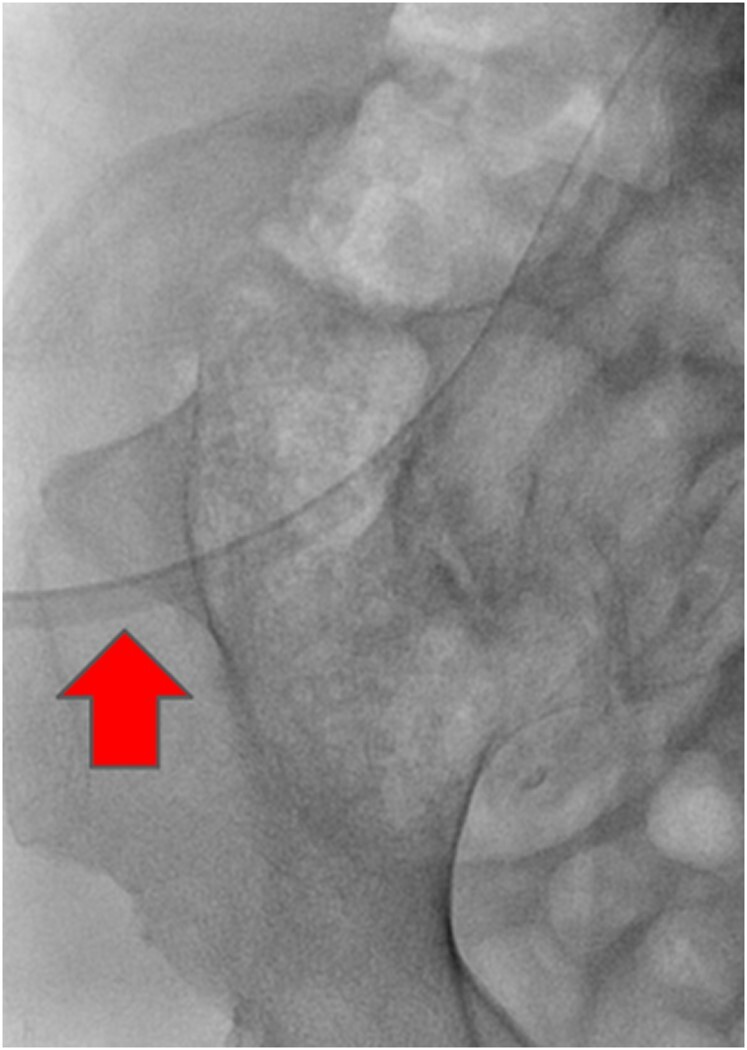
An anteroposterior right pelvic radiograph demonstrating the presence of a triangular osseous protrusion from the posterior aspect of the right ilia, the right iliac horn.
